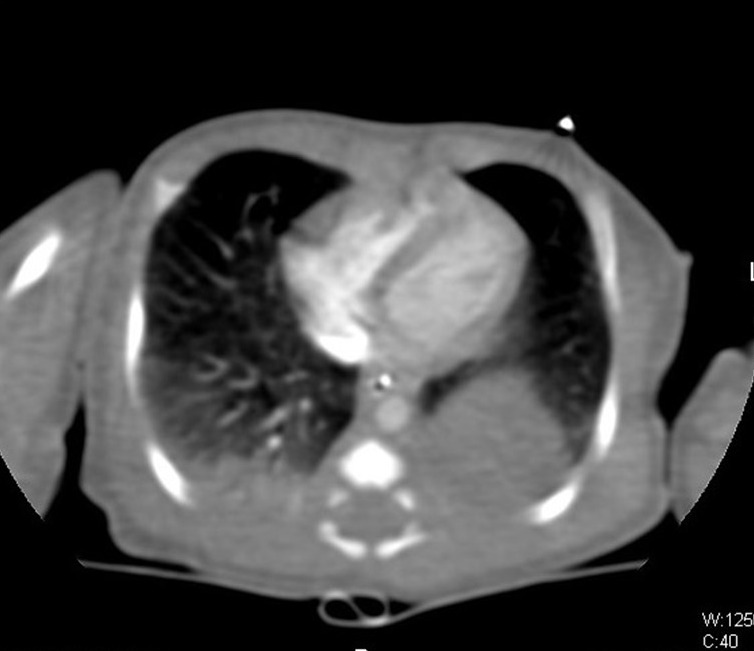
Figure 1:Chest CT showed intra-thoracic mass (extra-lobar sequestration) in the lower postero-lateral part of the left lung.
